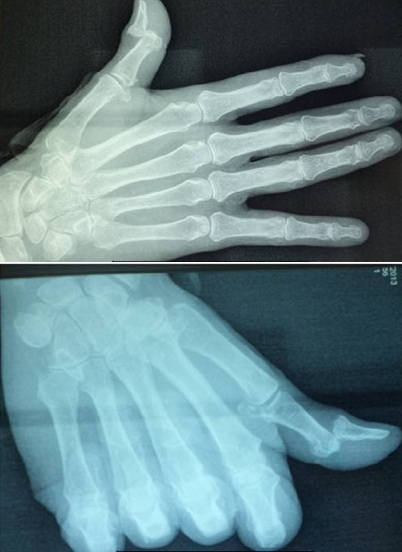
Anteroposterior and lateral radiographs display the dorsal dislocations of the interphalangeal joint
